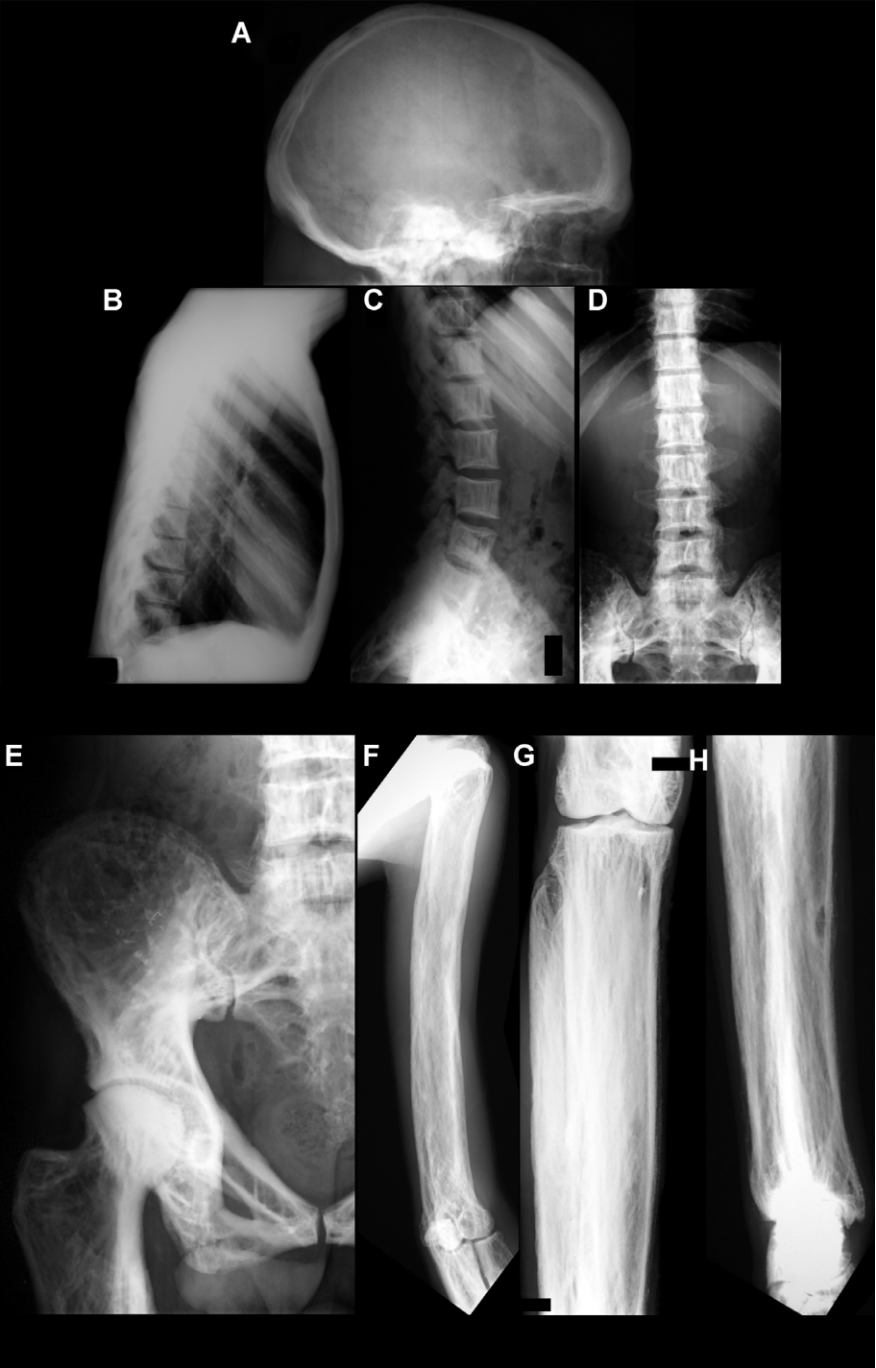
Radiographic findings of Patient 1 (son): Diffuse osteoslcerosis is present throughout the skeleton. (*A*) Lateral radiograph of the skull at age 32 years shows frontal calvarial thickening and sclerosis. The parietal, temporal, and occipital bones are largely spared. Normal intramedullary space is lost throughout the calvarium. (*B*) Lateral radiograph of the chest shows cortical and trabecular thickening throughout uniformly expanded ribs. Vertebral bodies are not enlarged but show similar thickening of the cortices and trabeculae and are diffusely radiodense. (*C,D*) Thickening of the cortex affects both the vertebral body and its posterior elements, but with minimal enlargement of the bodies and only moderate expansion of the posterior elements. A shared change affects the medullary space of the vertebral bodies and their posterior elements, producing coarse, thick, vertically oriented trabeculae in a pattern reminiscent of vertebral hemangiomas. (*E*) Anteroposterior radiograph of the right hemipelvis shows changes similar to those in the spine, with diffuse, mild bone expansion and cortical and trabecular thickening that follows normal stress line patterns, and are not as disorganized as seen in Paget bone disease. Marked cortical thickening and uniform sclerosis is particularly prominent in the superior and inferior pubic ramus, with no cortical splitting or “tram-tracking.” Cartilage loss and spurring in both hips indicates moderate osteoarthritis. (*F-H*) Anteroposterior radiographs of the upper (*F*) and lower extremities (*G,H*) show similar changes throughout the long tubular bones. There is diffuse bone expansion with cortical changes including cortical thickening, periosteitis that is more mature proximally than distally, and coarsening of the medullary trabecular pattern most prominent in the metaphyses and epiphyses. Bone shape is almost normal at the epiphyses, where there is no expansion or deformity. No significant arthritic change was noted in the shoulders, elbows, wrists, knees, or ankles. Notably, the periostitis extends to the ends of the long tubular bones in a pattern similar to primary hypertrophic osteoarthropathy (pachydermoperiostosis). Bone expansion also involves the short tubular bones of the hands with changes in the trabecular pattern resembling the long tubular bones (not shown).
